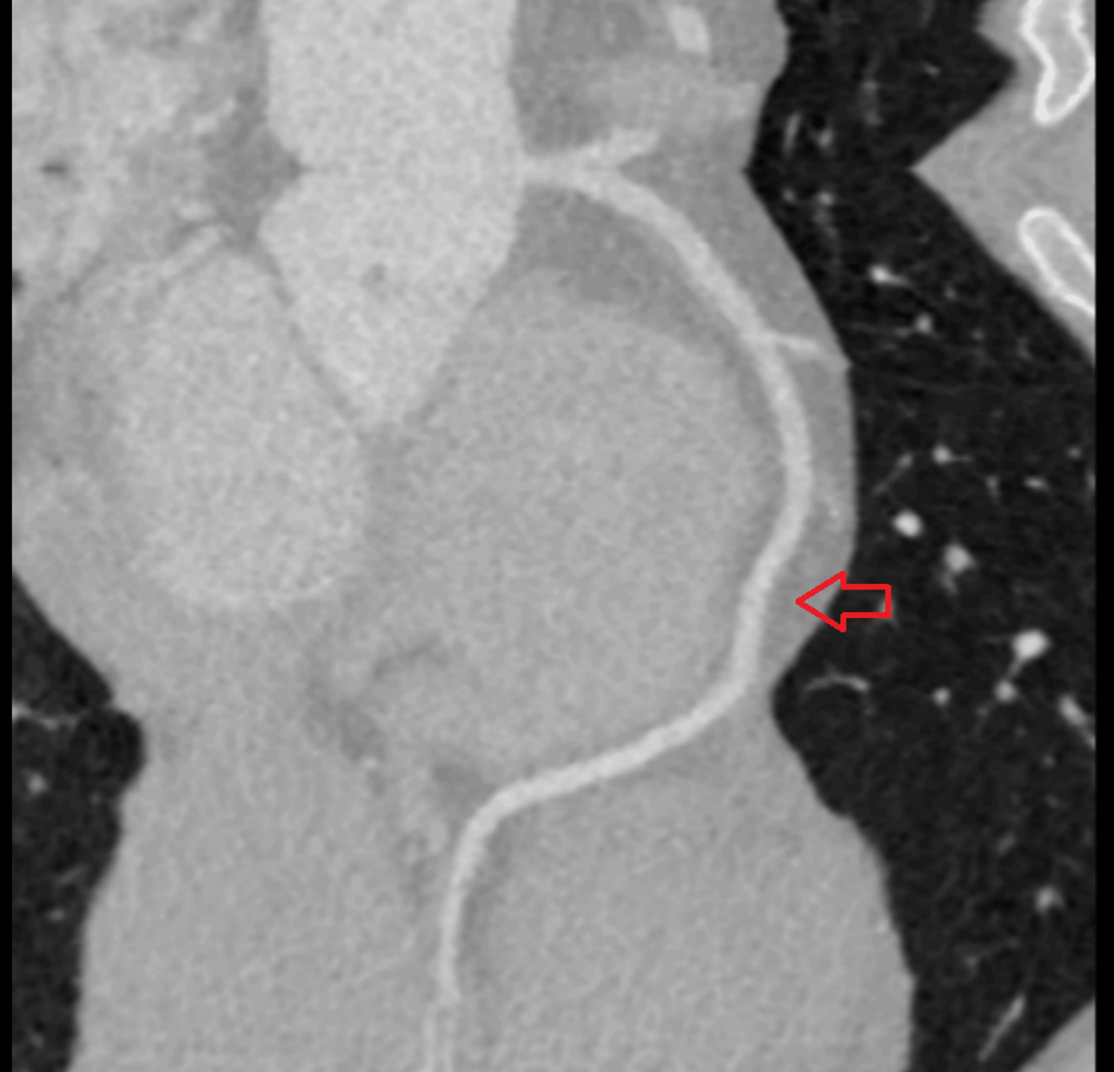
Coronary computed tomography angiogram revealing proximal left anterior descending artery myocardial bridging (shown by the red arrow)
